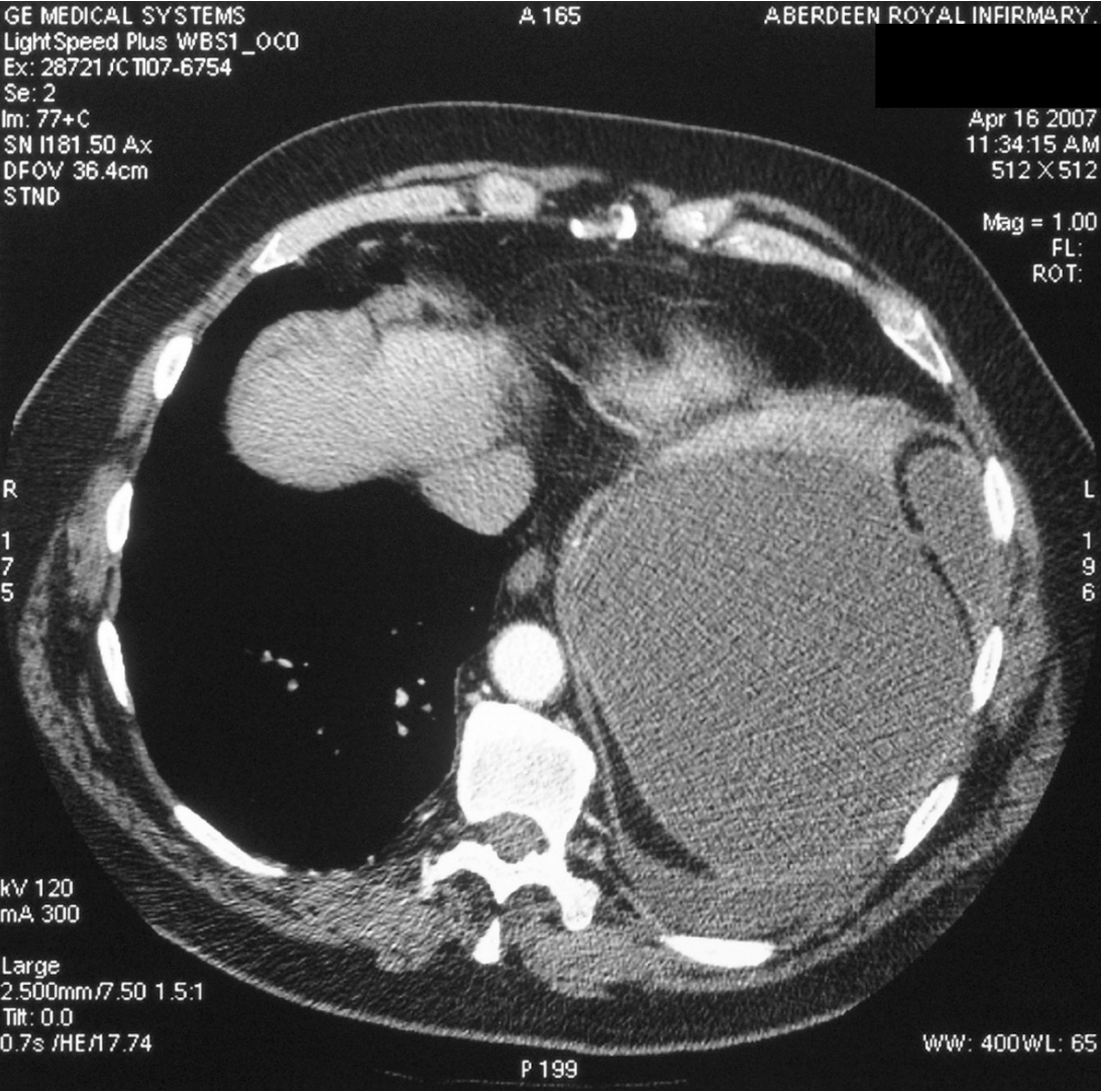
Computed tomography scan. Axial view of patient's CT scan showing a large peri-splenic haematoma.
